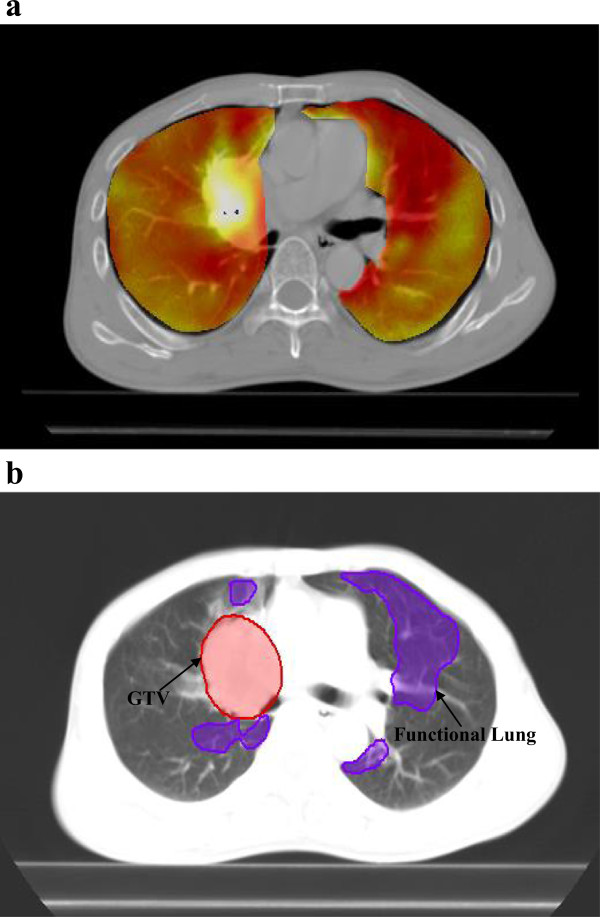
The functional lung of ventilation maps in a case. (a) Fusion imaging of CT image and pulmonary ventilation extracted from the generated deformation field; and (b) highly functional lung regions contoured on (a). In this study, regions in the top 30% for ventilation in the ventilation image were defined as functional lung structures to avoid in the functional IMRT plans.
